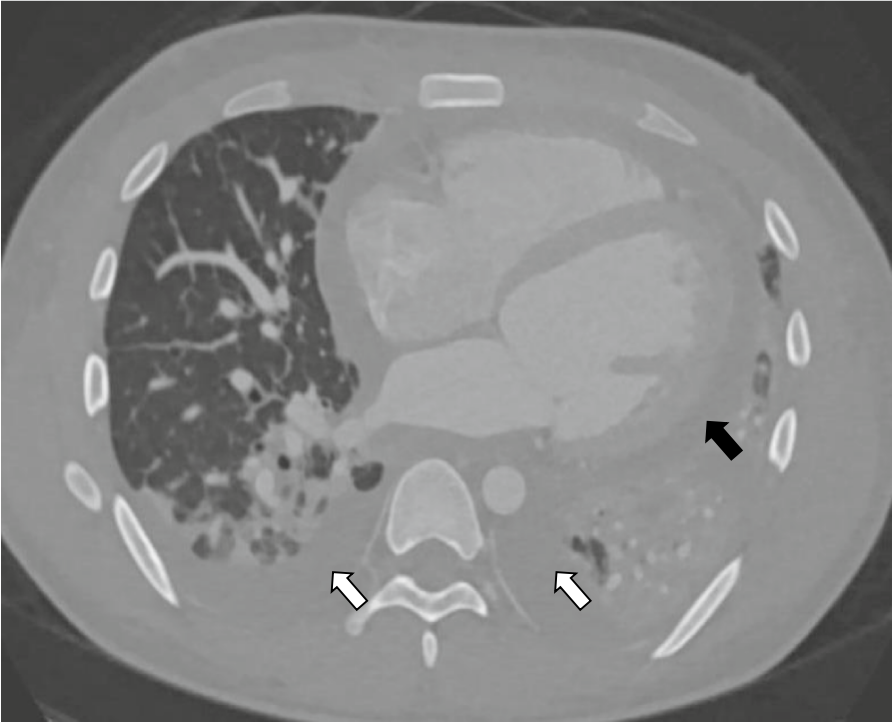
An axial view on computed tomography (CT) chest imaging obtained after the patient’s rapid cardiopulmonary decline demonstrating dense pulmonary consolidations, diffuse pulmonary nodules, and moderate pericardial (black arrow) and bilateral pleural effusions white arrows)
